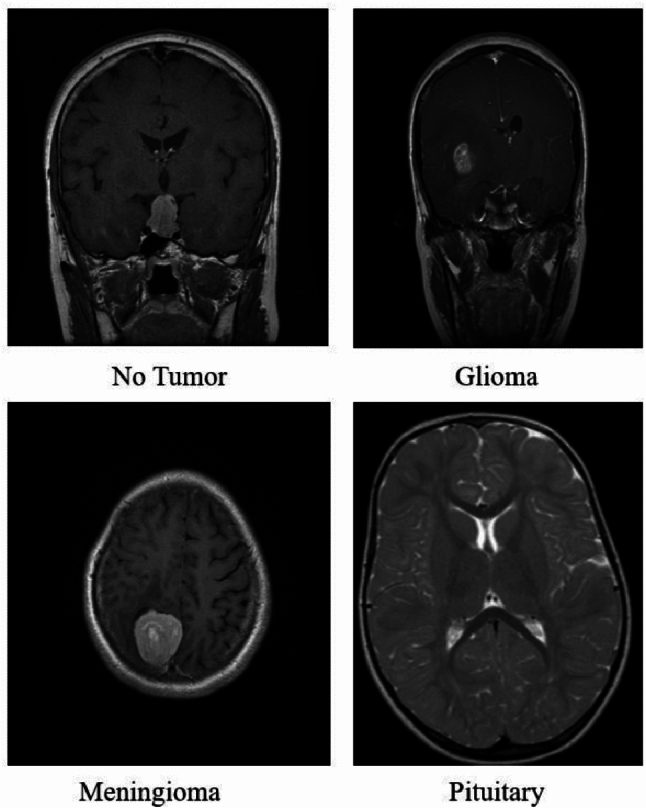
MRI scan indicating tumor^38^.
